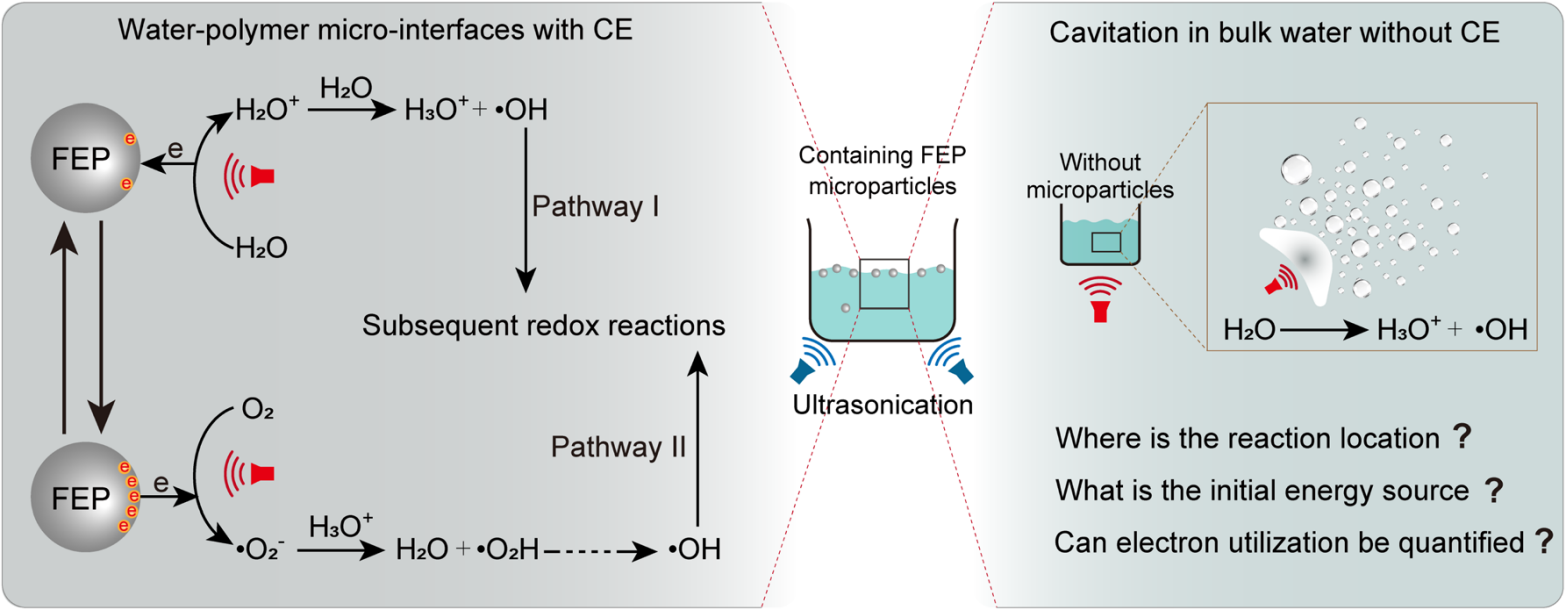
Reaction mechanism at the water/hydrophobic micro-interface driven by ultrasound (left) and possible reactions occurring in bulk water under intense energy input (right).
